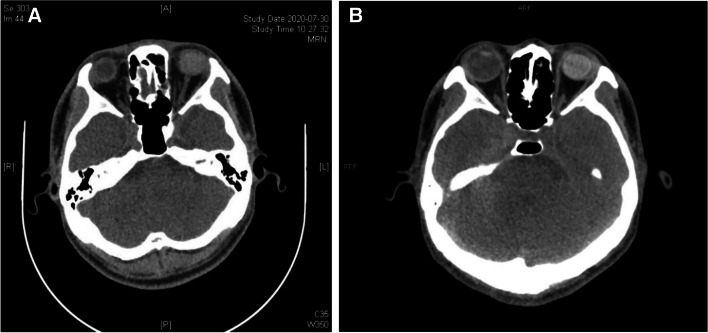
Postoperative follow-up results of FCVB implantation in case 20. A Preoperative CT. B CT 24 Weeks after operation
